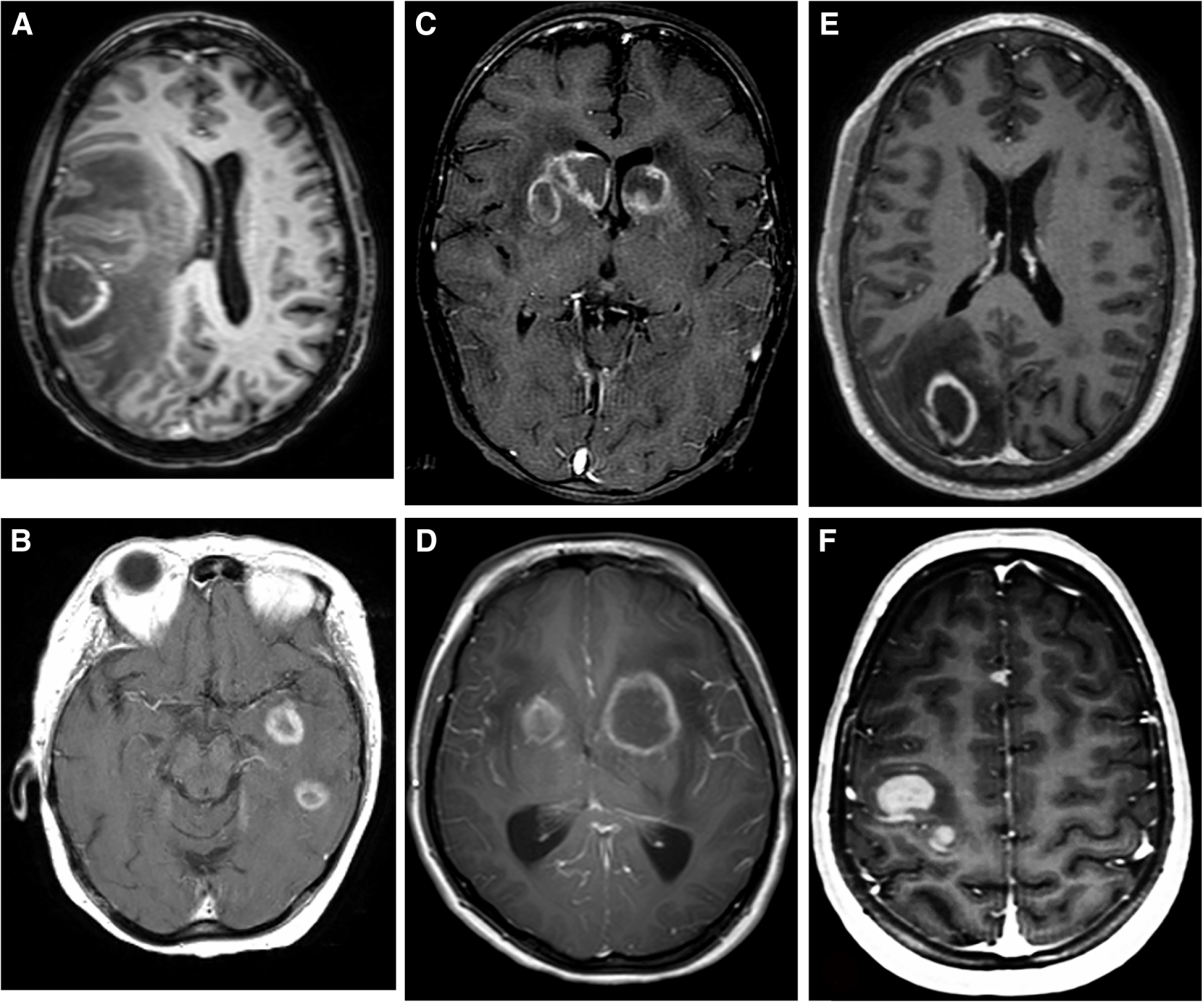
CNS-PTLD T1-weighted post-gadolinium images in six cases (a-f). In these cases, the lesions are predominantly ring enhancing. Although, case f has two solid enhancing lesions. There are no solid enhancing periventricular lesions as typically seen in CNS lymphoma. The location of lesions in the basal ganglia in cases c and d would be unusual for metastatic disease
